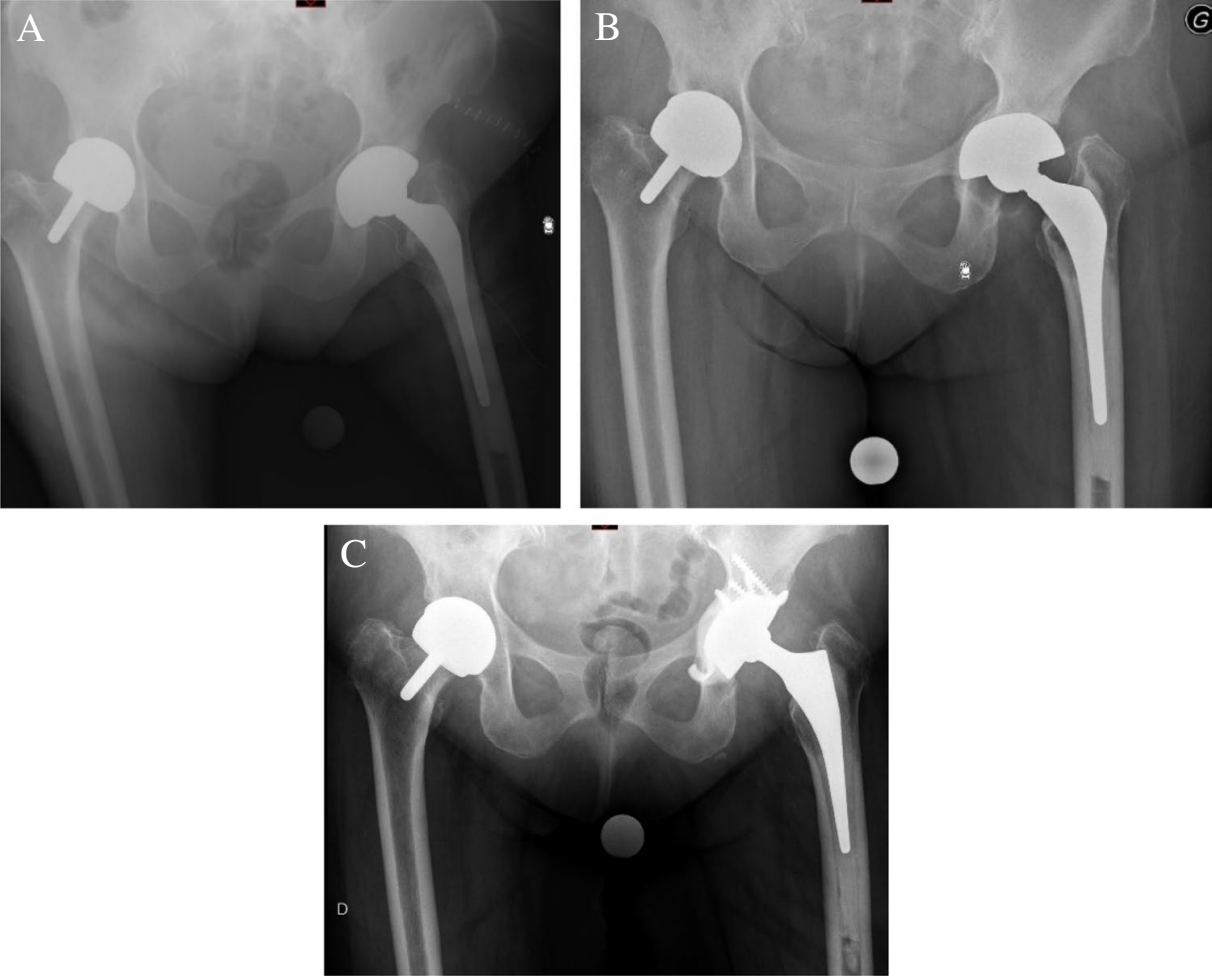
Case of a 92-year-old male patient presenting cup migration, which required revision surgery. A Postoperative antero-posterior radiograph of the pelvic with revision using a cementless dual mobility cup. B At 6-week follow-up, pelvic radiograph showed aseptic migration of the dual mobility cup. C After re-revision using a Ganz reinforcement ring with a cemented dual mobility
